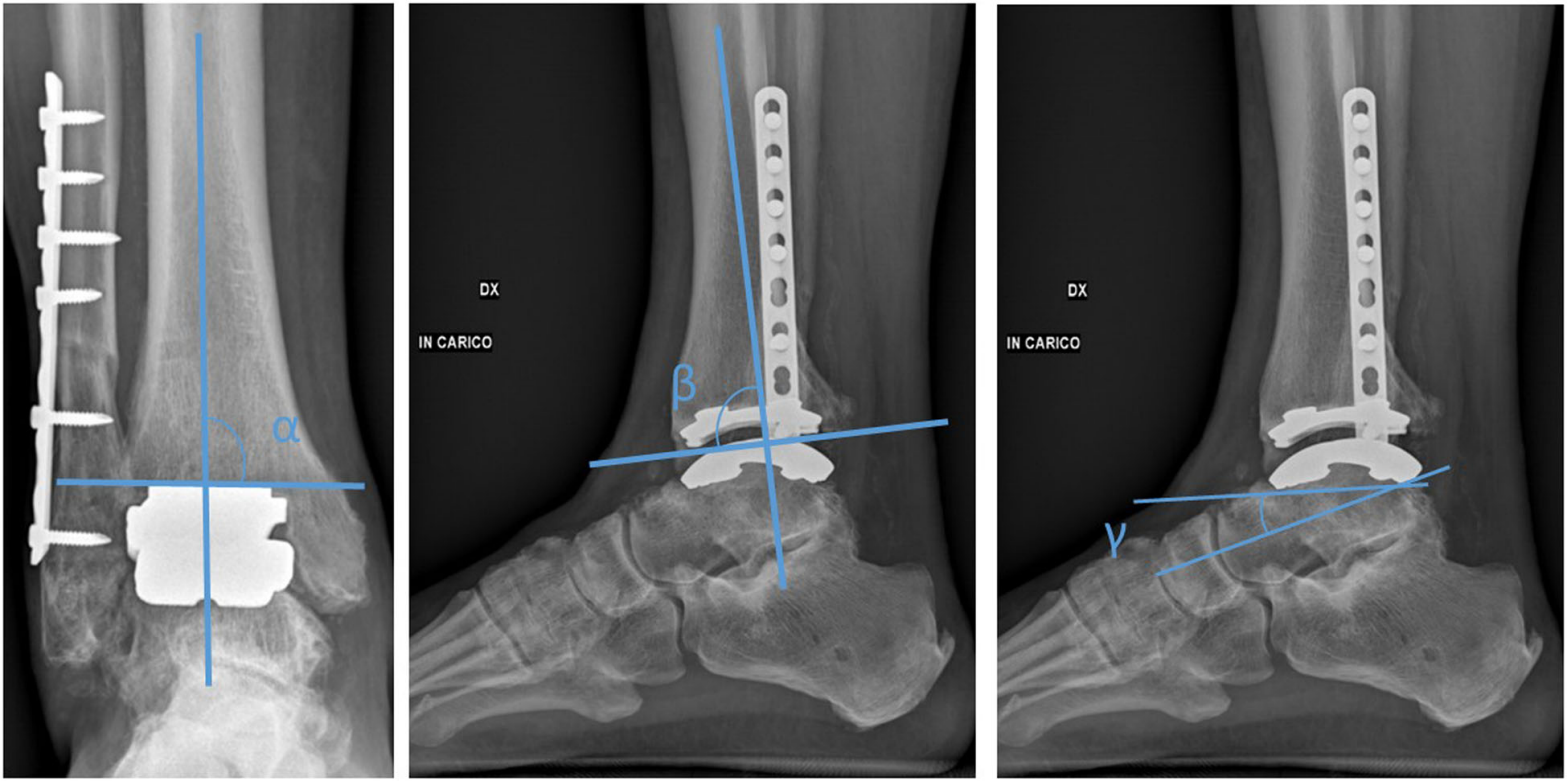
Radiographic angles (α, β, γ) for assessment of components position and migration
